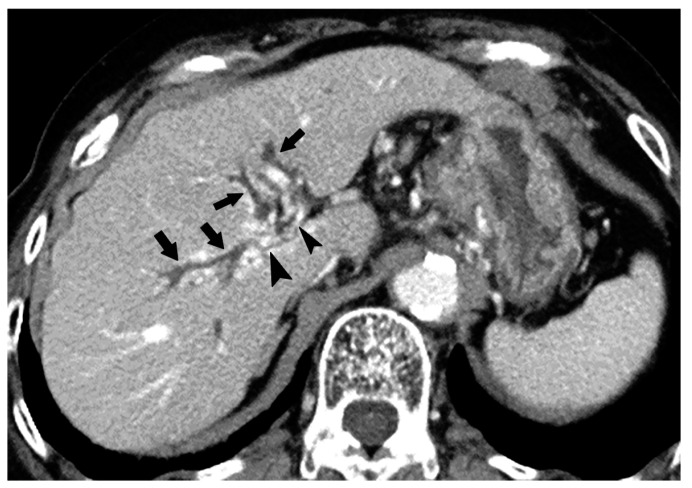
Intrahepatic portal vein thrombosis in an 84-year-old woman. In the portal venous phase of contrast-enhanced CT, the portal vein in the hepatic hilum shows linear hypodensity (arrows) and looks like bile duct dilatation. However, there are markedly enhanced multiple vessels (arrowheads) around the hypodense linear structure, which is called cavernous transformation of the portal vein. It is a typical finding of portal vein thrombosis in the hepatic hilum area.
